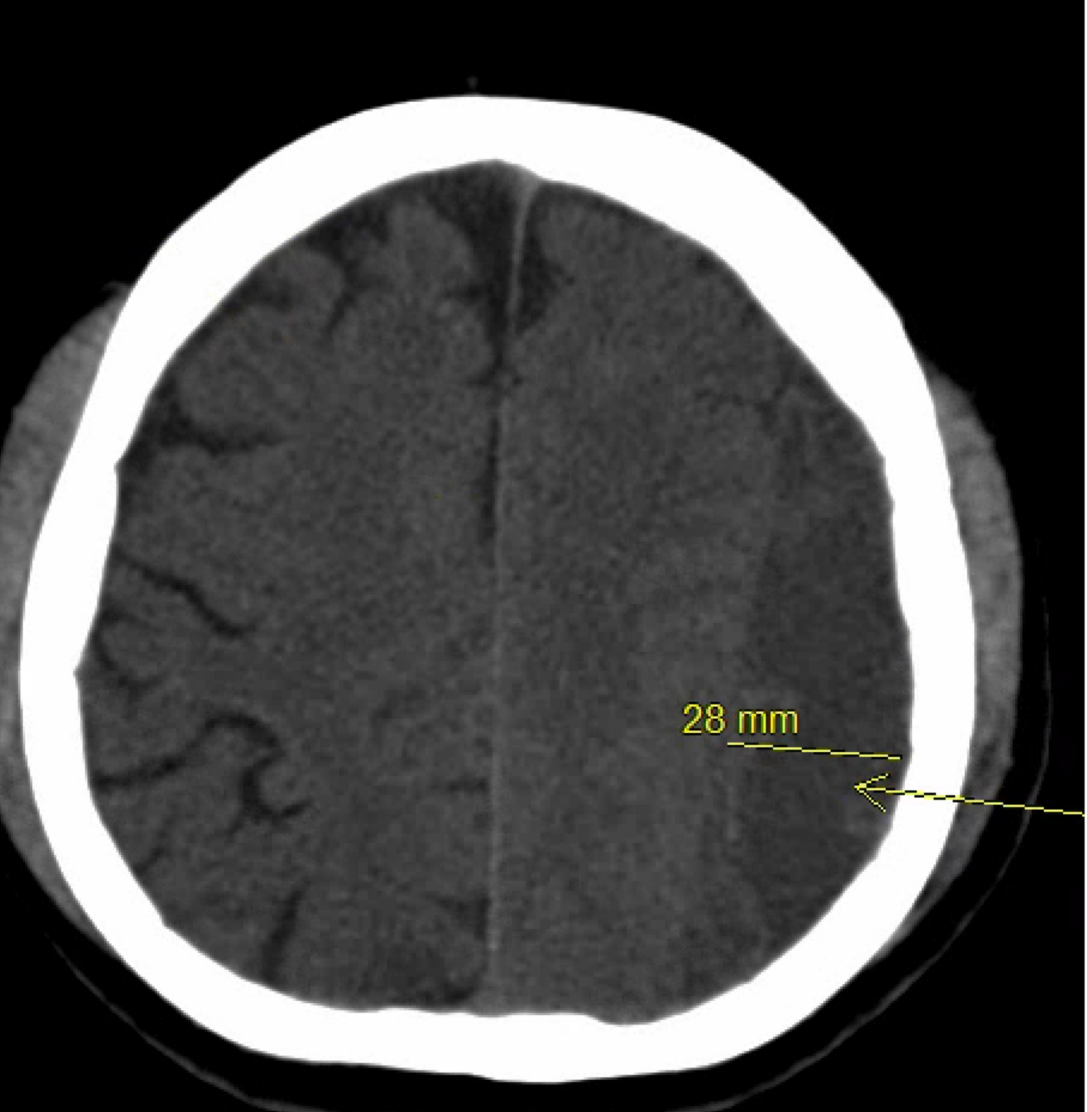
Pre-drainage CT scan of a patient who underwent subdural drain placement with tissue plasminogen activator adjunct Thickness was measured at approximately 28 mm; the arrow is pointing to the location of the subdural hematoma providing significant mass effect.
